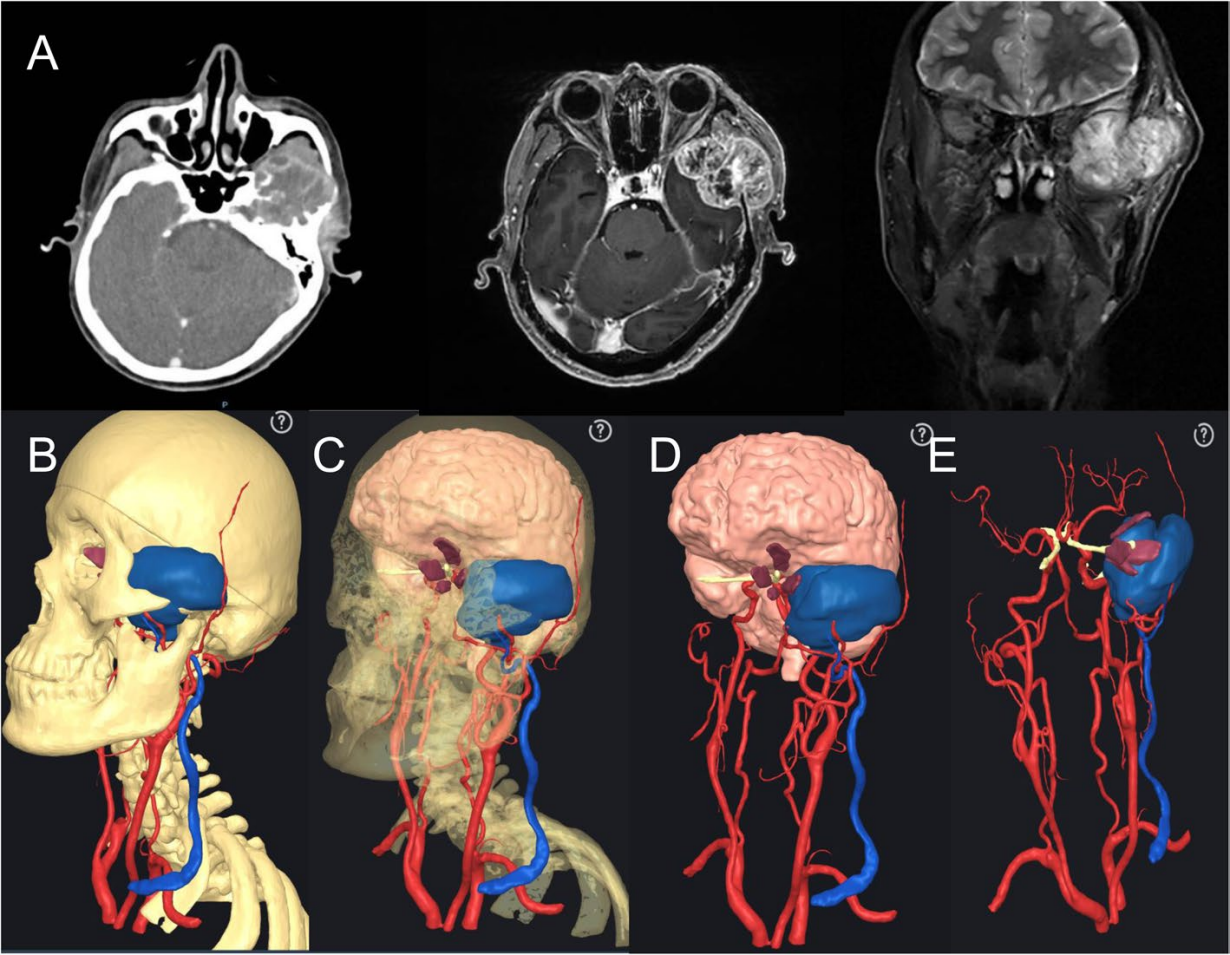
A Preoperative enhanced CT and MRI imaging showed the extent of the lesion. B Three-dimensional reconstruction after skin and soft tissue removal. C The relationship between the tumor and adjacent tissues was demonstrated by crypto jaw enucleation and skull transparency. D Saphenous osteotomy showed tumor and important structures (only blood vessels, brain, and nerves). E The tumors and important structures (only blood vessels and nerves) can be shown in the brain tissue
